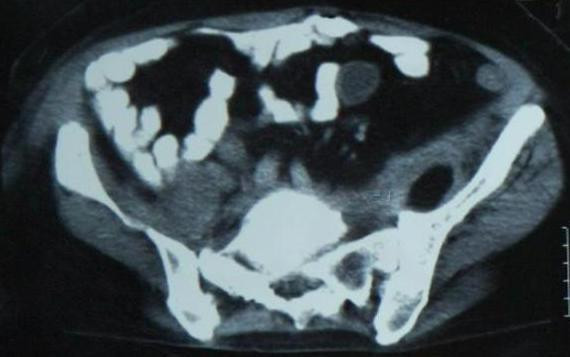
In the abdominal CT, no abscess observed in the right and left psoas muscles.
